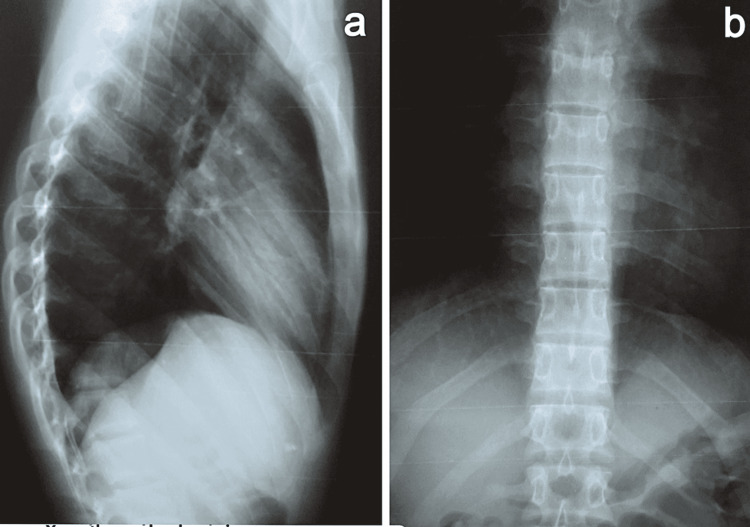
Thoracolumbar spine x-ray. (a) Lateral view. (b) Anteroposterior view.
